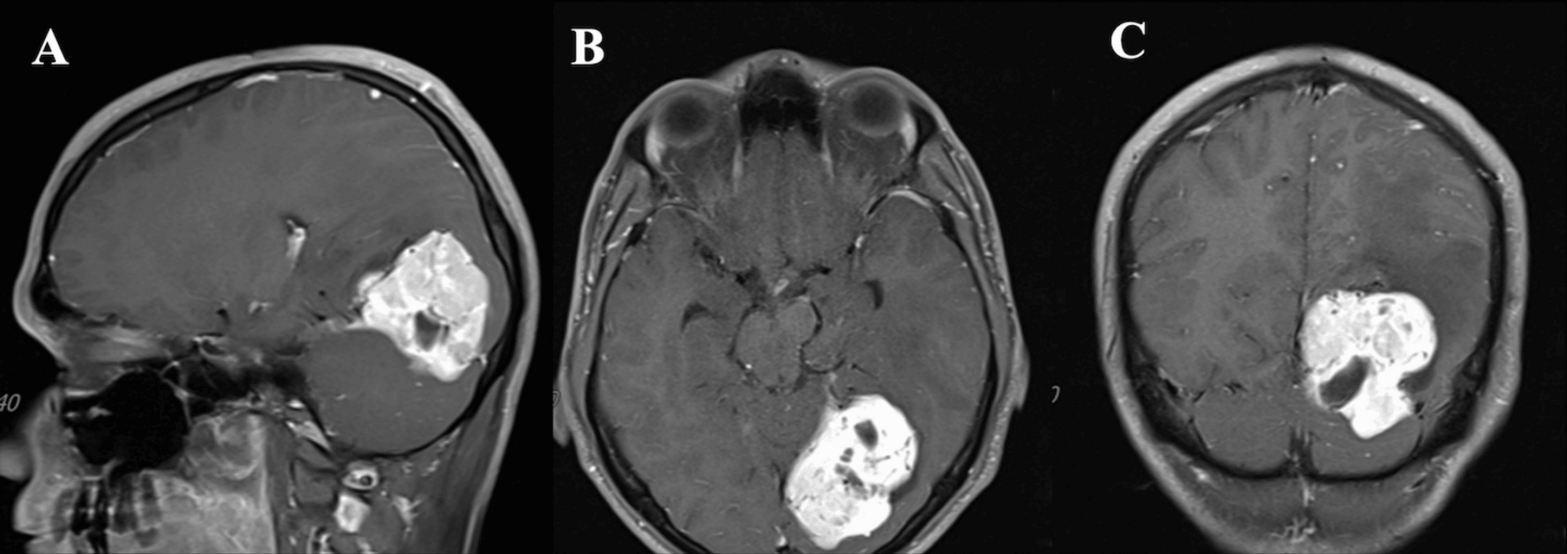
Preoperative brain MRI. Sagittal (A), axial (B), and coronal (C) gadolinium-enhanced T1WI showing a large tumor in left occipital region, originating from the falx, extending into the tentorium, and exhibiting heterogeneous enhancement.
